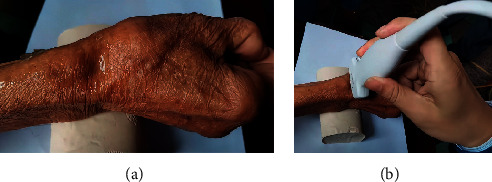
(a) Anatomic snuffbox area in one patient with low body mass index. (b) Ultrasound follow-up operation.
